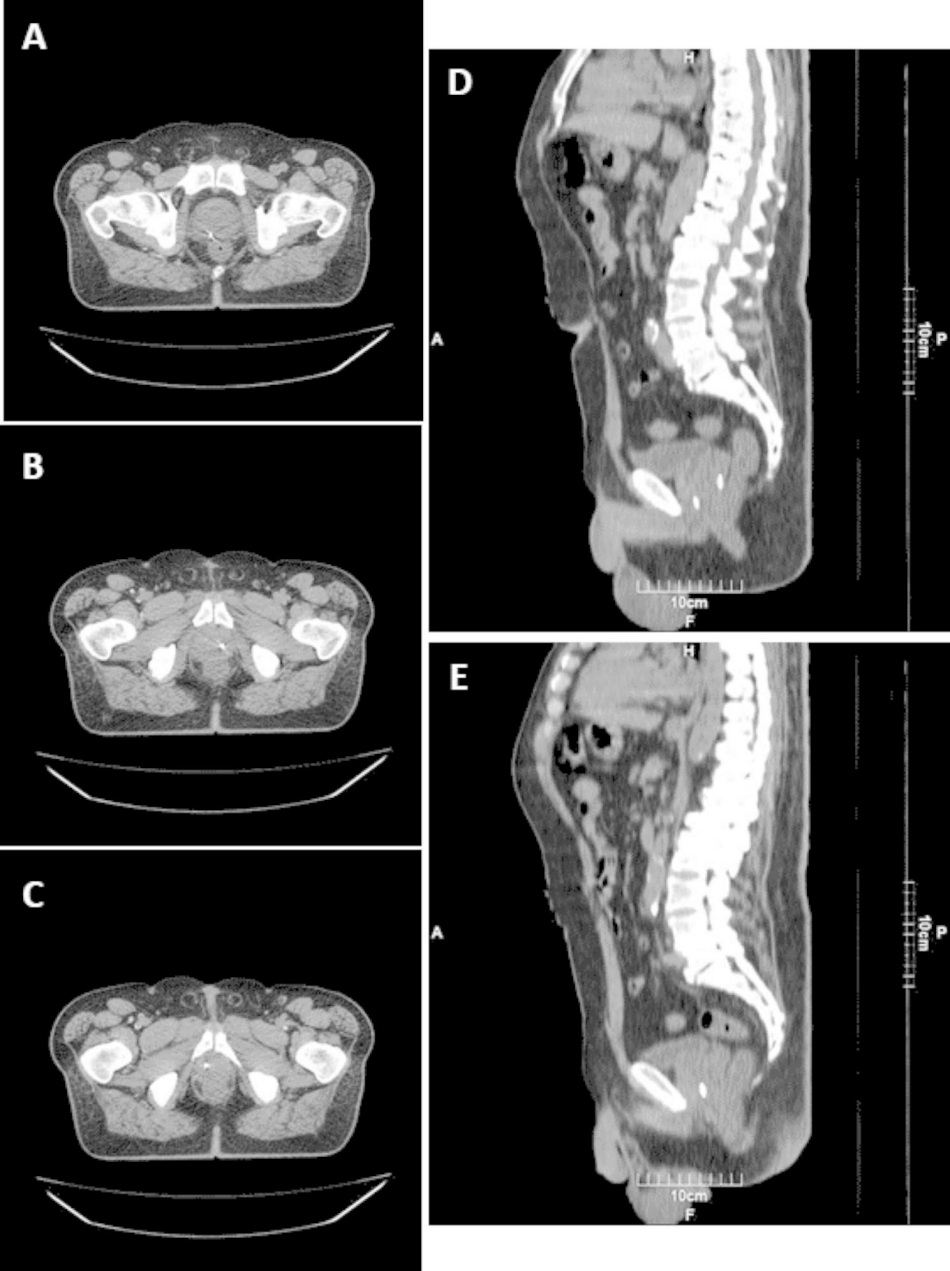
Fiducial gold marker insertion in a patient with confirmed prostatic adenocarcinoma candidate for IGRT. Three fiducial markers were inserted in different parts of the prostate gland under TRUS guidance, and the position was confirmed with a CT scan performed for planning. Two fiducial markers were placed close to the prostatic lesions (white arrows in Fig. 3)
